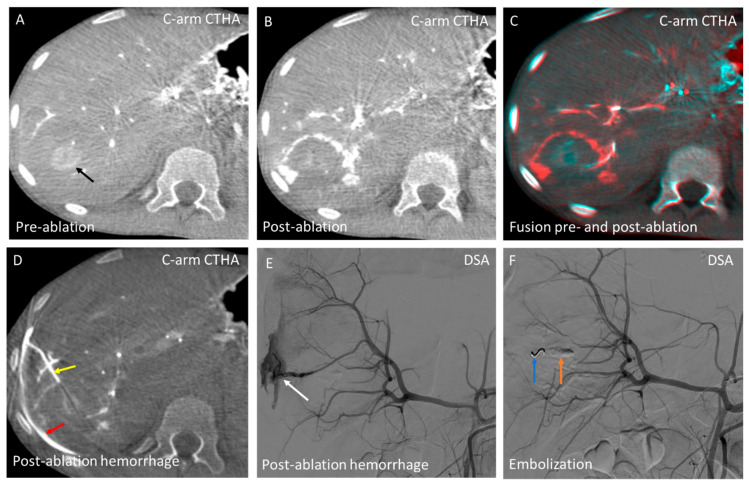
A 13-year-old patient with severe post-ablation hemorrhage requiring immediate embolization. (A) The target lesion (black arrow) (20 mm) is detected on intraprocedural C-arm CT hepatic arteriography (C-arm CTHA); (B) Post-ablation C-arm CTHA depicts the ablation zone after 3.5 min at 150 W; (C) Axial fusion of pre- and post-ablation C-arm CTHA shows adequate ablation margins; (D) Post-ablation arterial hemorrhage is observed in the ablation needle tract (yellow arrow) with subcapsular bleeding (red arrow); (E) Digital subtraction angiography (DSA) reveals contrast extravasation (white arrow), requiring immediate embolization; and (F) Bleeding was resolved within 8 min from onset with coil (blue arrow) and gelfoam embolization (orange arrow). Post procedure, the patient remained hemodynamically stable with only temporary complaints of shoulder pain.
